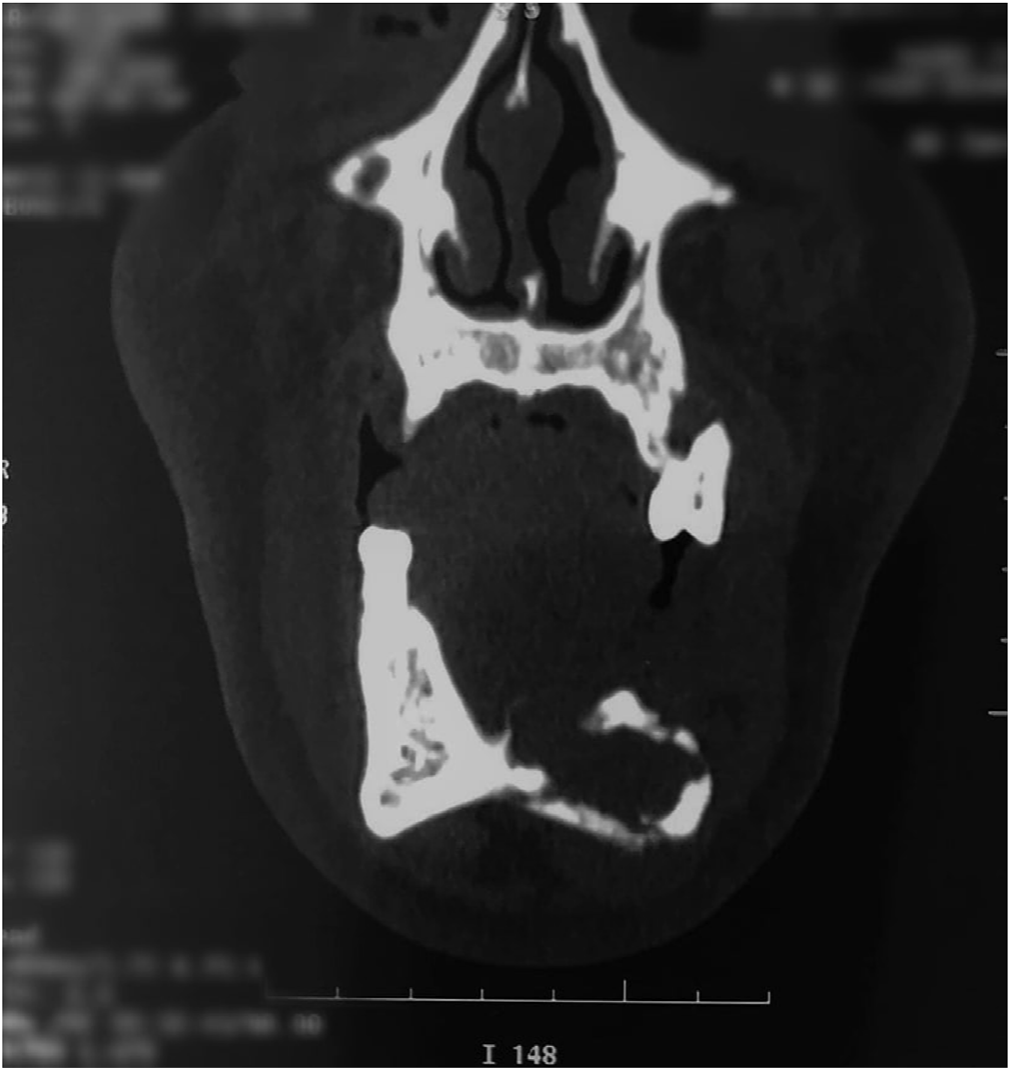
CT scan with frontal section showing an osteolytic mandibular lesion with a maxillary floating tooth.
